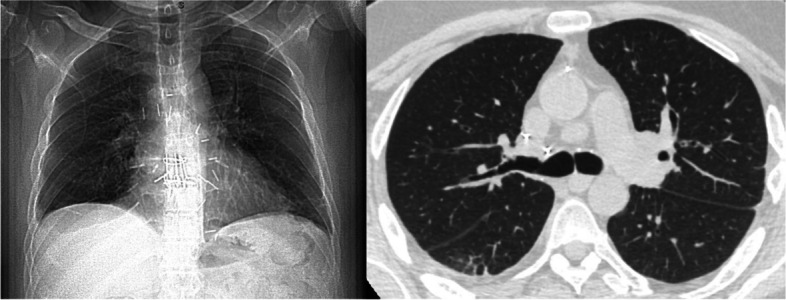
Chest roentgenogram and chest computed tomography after discharge. The pneumatocele has disappeared
